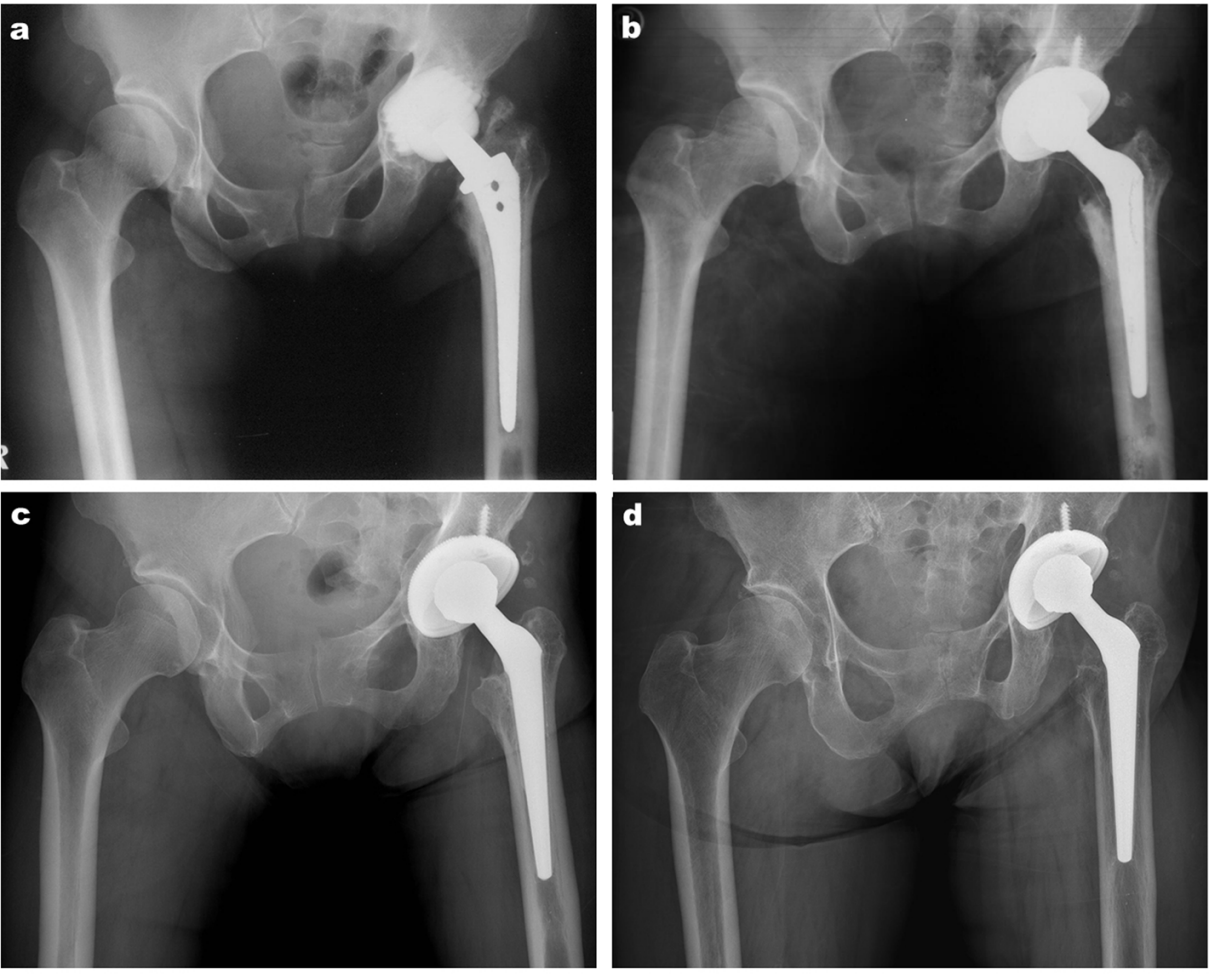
a Preoperative radiograph of a 57-year-old woman with aseptic loosening of the Autophor femoral stem. b Immediate postoperative radiograph of the left hip: revision was performed using the Wagner cone stem and cementless acetabular cup. c Anteroposterior radiographic view of the left hip 3 years after the revision total hip arthroplasty (THA). d Anteroposterior radiographic view of the left hip 20 years after the THA shows grade II stress shielding in Gruen zones 1 and 7
